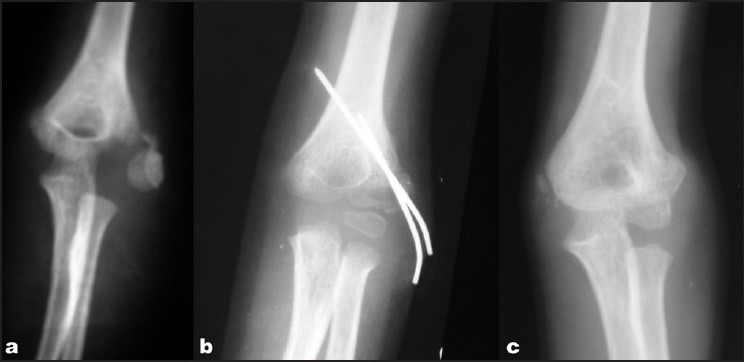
X-ray of the elbow joint (anteroposterior view) showing (a) eight-week-old fracture of the lateral condyle of the humerus. (b) Immediate postoperative X-ray after fixation with two K-wires; (c) same patient after union
